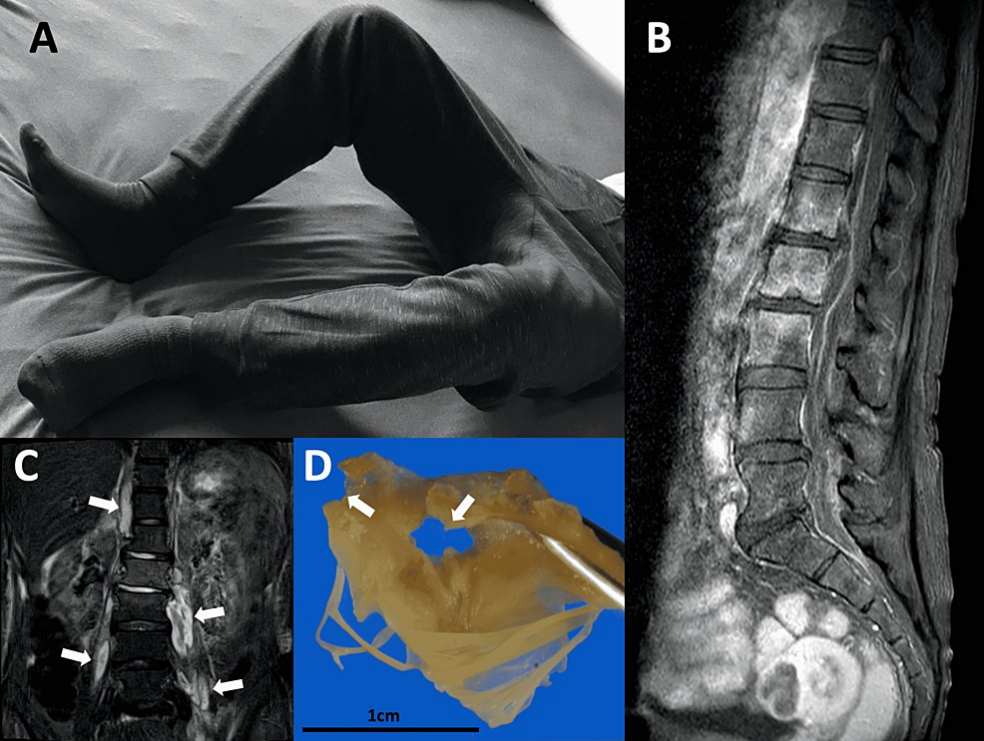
Multiple diagnostic images A) An example of “psoas position”. The patient remains in a hip-joint flexion position. This is a reproduced view of the actual patient’s limb position. B) Magnetic resonance imaging (MRI) enhanced T1 image of the spine. L1-L3 area shows purulent spondylitis. C) MRI enhanced T1 image of the bilateral iliopsoas abscess (arrows). D) The resected mitral valve disrupted by infected valvulitis. Arrows indicate the perforations of the valve.
